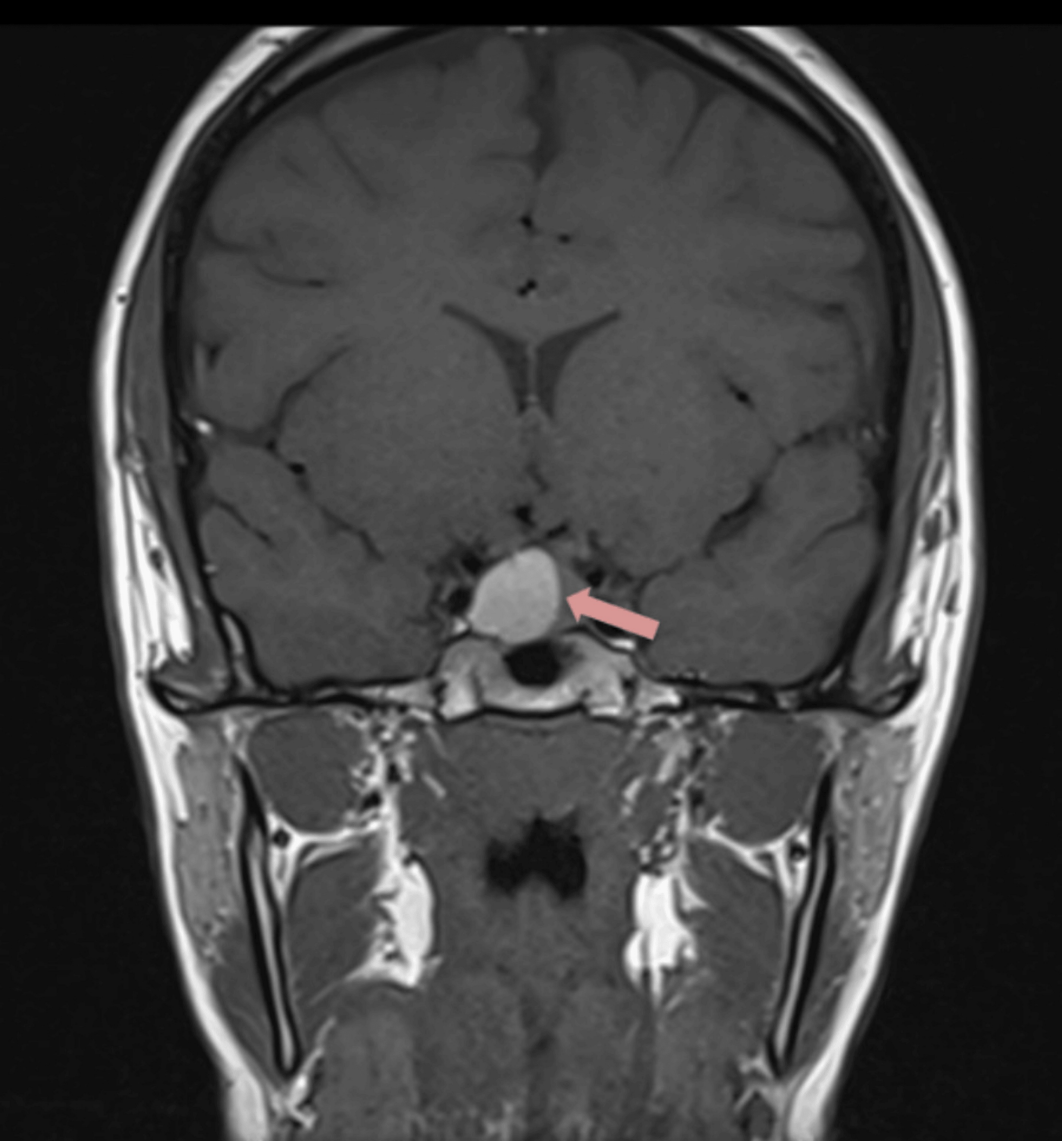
Contrast-enhanced pituitary magnetic resonance imaging (MRI) demonstrating pituitary apoplexy (arrow). Dedicated pituitary MRI with contrast shows a 12 × 13 × 15 mm cystic sellar lesion with suprasellar extension and mild superior displacement of the optic chiasm. The lesion demonstrates a fluid-fluid level and hemorrhagic signal characteristics without significant post-contrast enhancement. The pituitary stalk is deviated leftward, the cavernous sinuses are unremarkable, and internal carotid artery flow voids are preserved.
